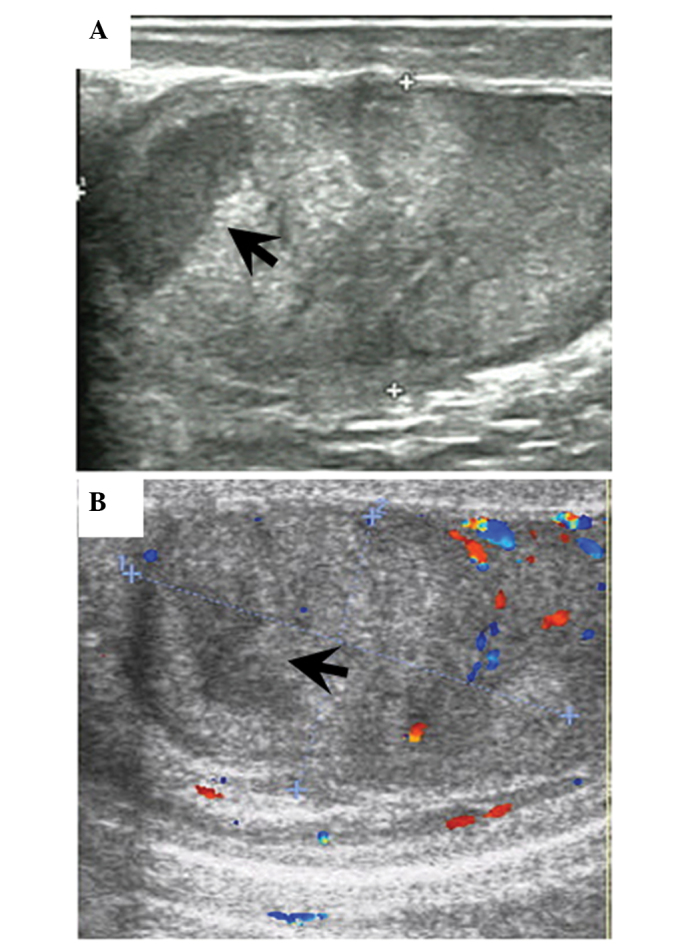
(A) Scrotal sonography demonstrated a wedge-shaped hypoechoic lesion located in the upper pole of the testis (arrow). (B) Color Doppler sonography revealed that the hypoechoic lesion was completely flowless (arrow).
